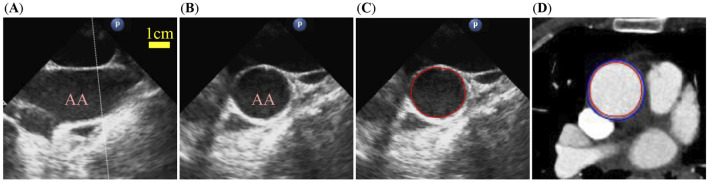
Medical images and segmentations of the ascending aorta. (A) Long-axis view of the TEE image. (B) Short-axis view of the TEE image at the position of the white dotted line in (A). AA, ascending aorta. (C) Perimeter of the lumen marked in the TEE image. (D) Lumen and wall contours marked in the CT image.
